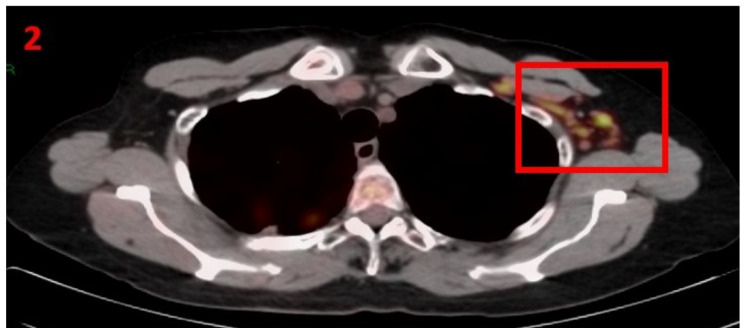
[18F]FDG PET-CT showing hypermetabolic uptake in the 2nd patient with lymphadenopathy in the left axillary region (red square).
